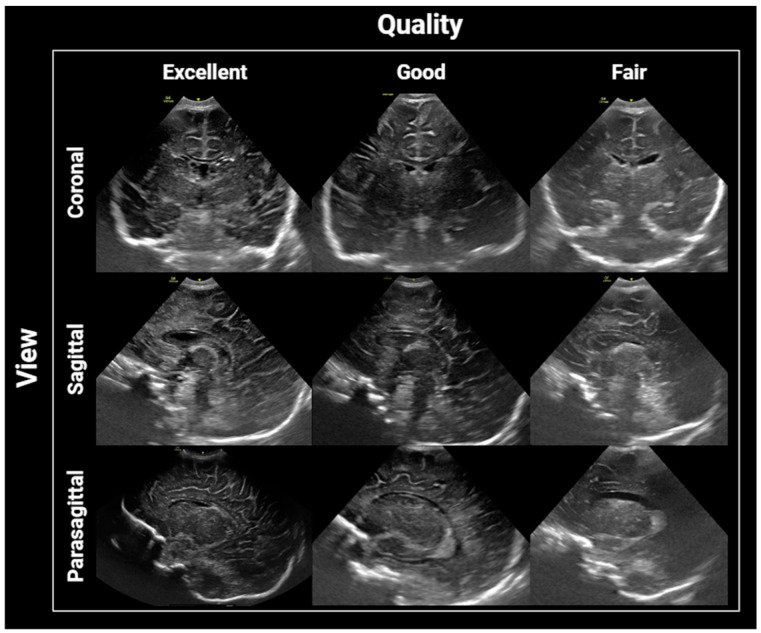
Quality grading of brain ultrasound studies in coronal, sagittal, and parasagittal views.
